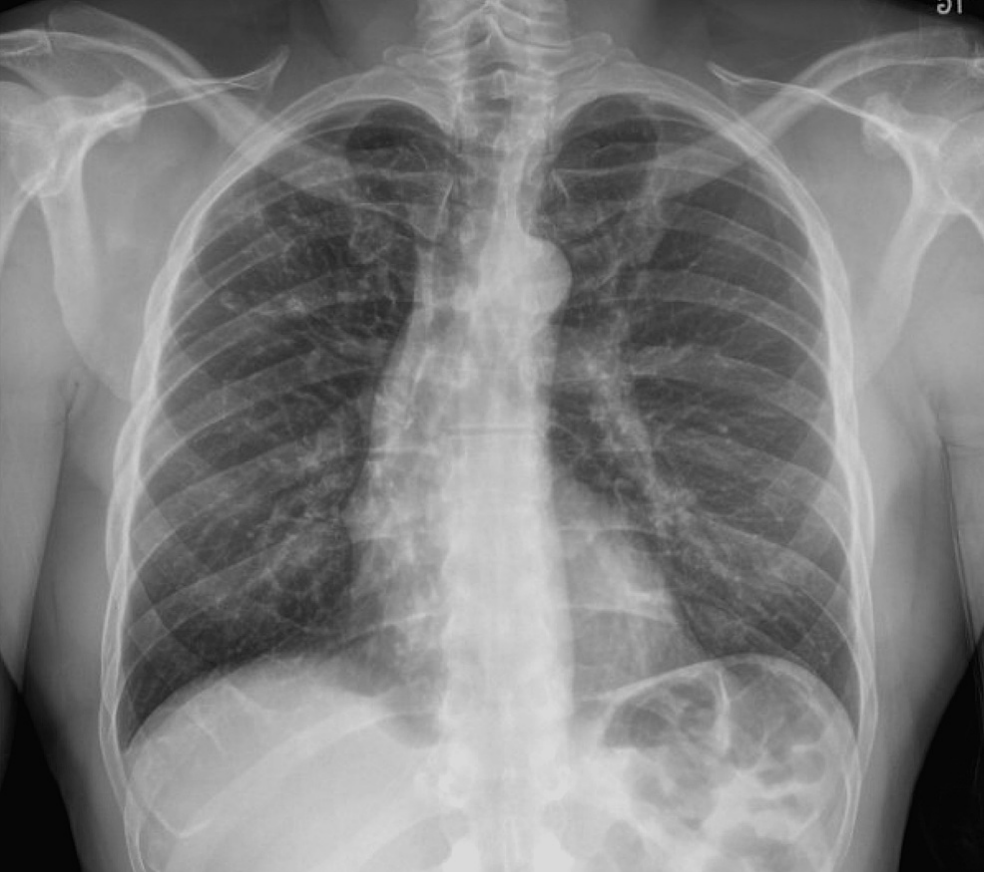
Unremarkable Chest X-ray
